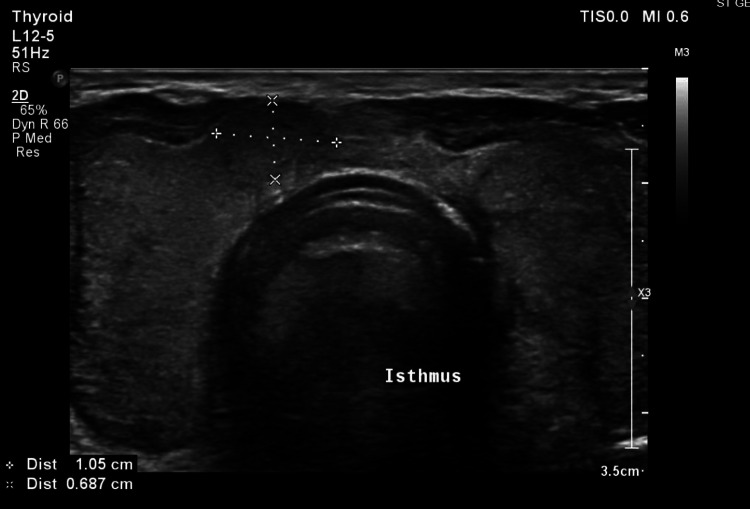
Ultrasound showing the enlarged heterogeneous thyroid with right lobo-isthmic nodule.
